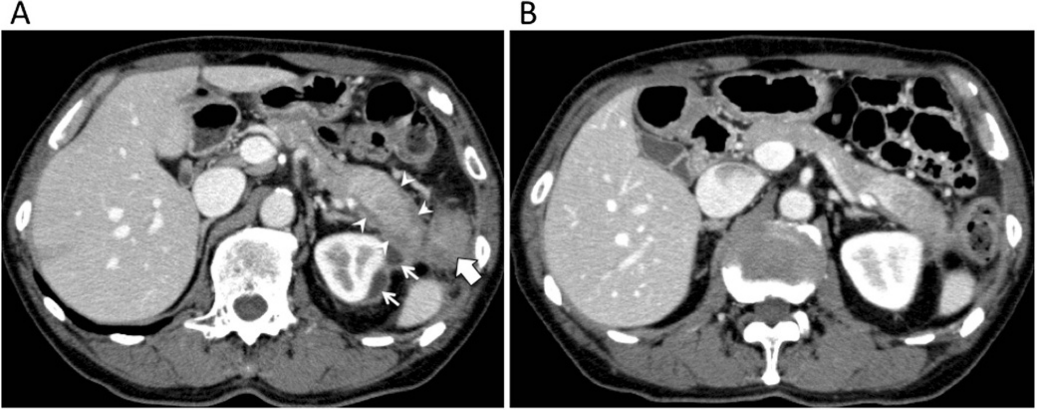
Enhanced computed tomography showing an enlarged pancreatic tail, before (A) and after (B) steroid initiation. A swollen pancreas, adhesive to the descending colon (large arrow), with a capsule-like rim (arrow head) and effusion (small arrow) around the left kidney (A). Minimized pancreatic swelling and decreased effusion (B).
